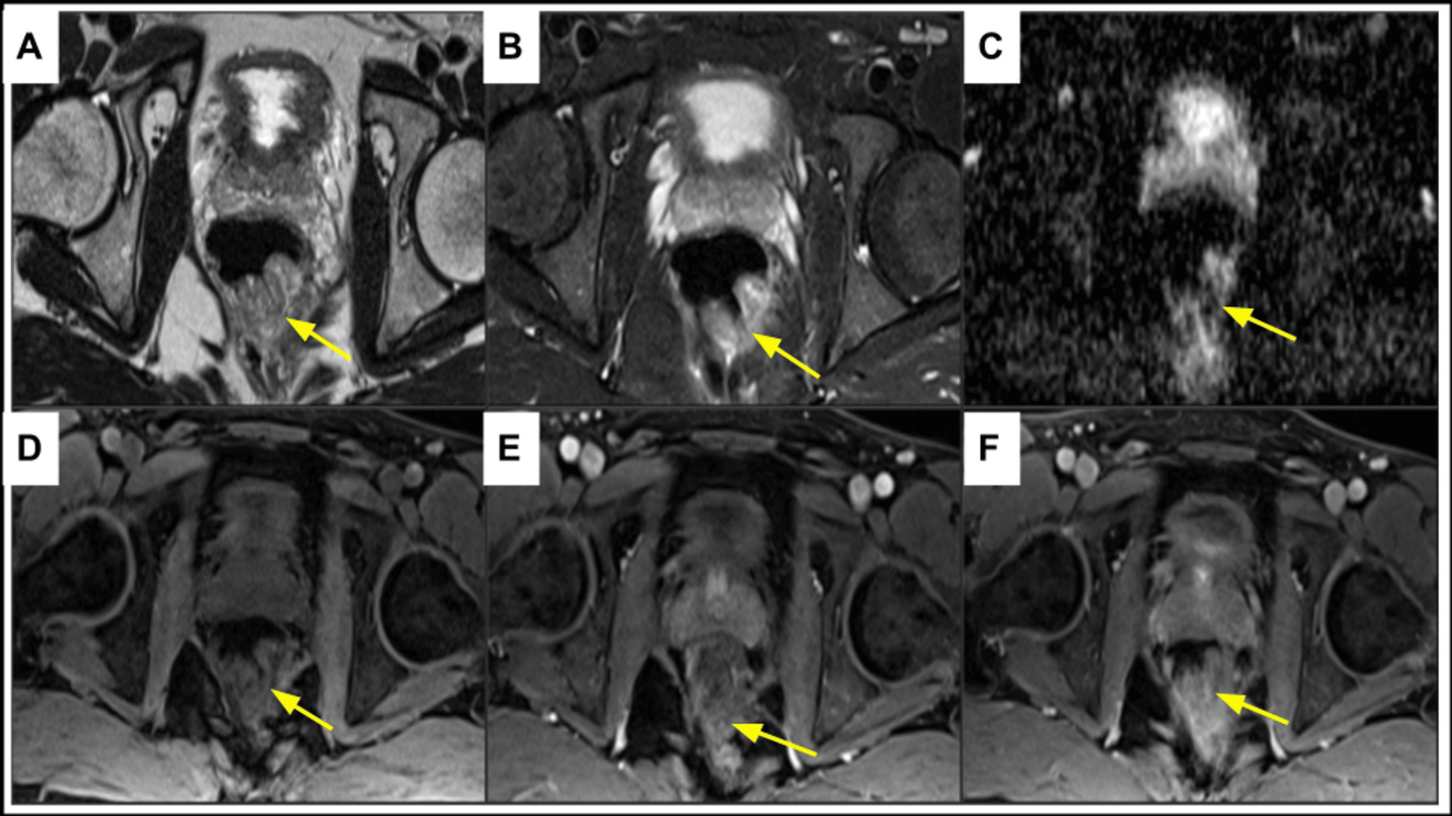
Magnetic resonance imaging of the pelvis demonstrates heterogeneous predominantly hyperintense rectal wall lesion (yellow arrow) on T2-weighted non-fat-saturated (A) and T2-weighted fat-saturated (B) imaging sequences. The lesion demonstrates restriction on diffusion-weighted (C) images. T1-weighted pre-contrast (D), post-gadolinium arterial phase (E), and post-gadolinium delayed phase (F) images demonstrate intense contrast enhancement of the rectal lesion. This lesion is consistent with biopsy-proven rectal schwannoma.
